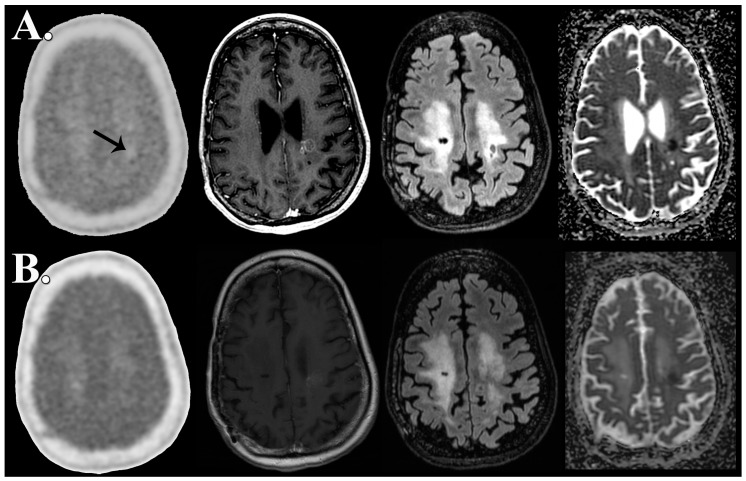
Abseent FMISO hypoxic volume in pseudoprogression prior to and following bevacizumab therapy. FMISO PET/MR imaging obtained simultaneously 7 days prior to (A) and 155 days after initiation of antiangiogenesis therapy (B) demonstrates pseudoprogression characterized by decreased volume of contrast enhancement (middle left) and nonenhancing FLAIR hyperintense mass (middle right) with associated focus of reduced diffusion (adc map, right). Unprocessed (left) and fused FMISO PET imaging (middle left, middle right, and right) demonstrates absence of FMISO accumulation above 1.2 time background. Pre-therapy FMISO PET/MR demonstrates slightly decreased focus of radiotracer uptake (upper left, arrow) relative to background which slightly increases to background uptake levels following bevacizumab therapy. FMISO HV is not observed at any time point in this patient with pseudoprogression.
